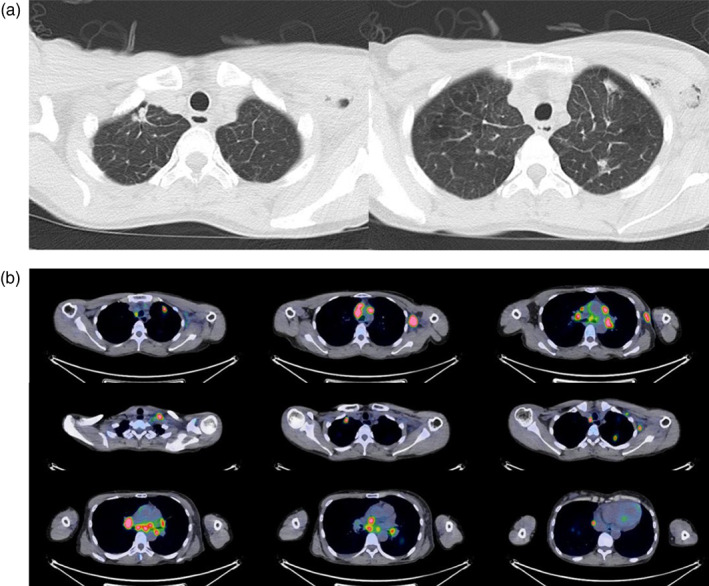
Imaging at initial diagnosis by (a) chest computed tomography (CT) and (b) positron‐emission tomography (PET)‐CT.
